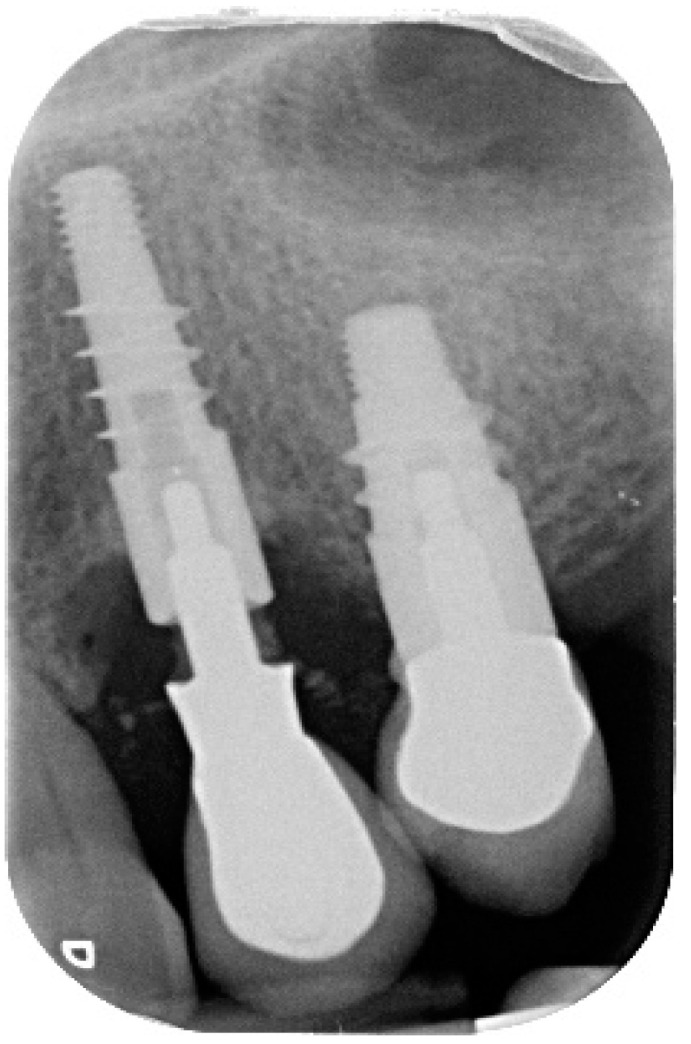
Pre-operative endoral X-ray.
